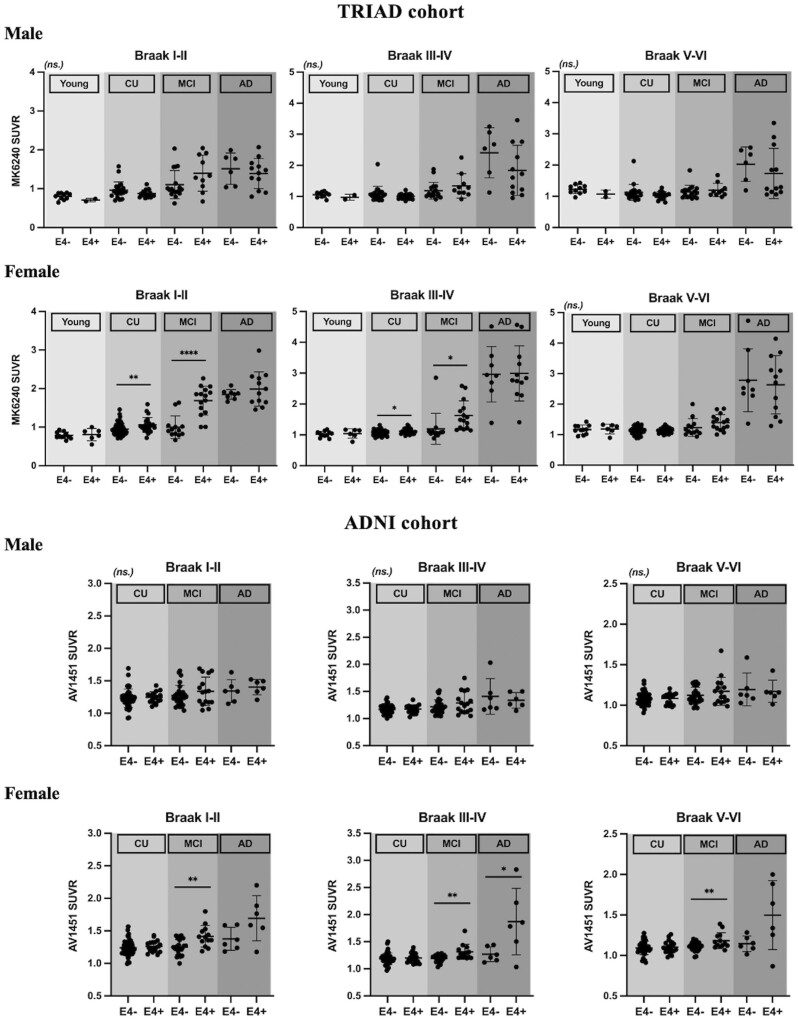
Tau-PET SUVRs were elevated in female *APOE*ε4 carriers with mild cognitive impairment in comparison to *APOE*ε4 non-carriers.
